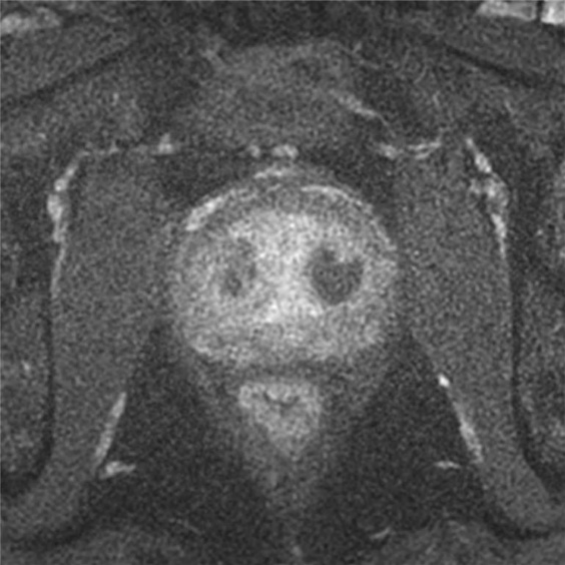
Three-month post-treatment MRI in a 69-year-old patient with concomitant bilateral treatment for prostate adenocarcinoma, Gleason 6 (PSA level of 8.14 ng/ml, 1/12 positive biopsy at right apex). Axial T1 FS contrast-enhanced image (a) shows the persistence of two small areas of residual necrosis (*arrowhead*)
